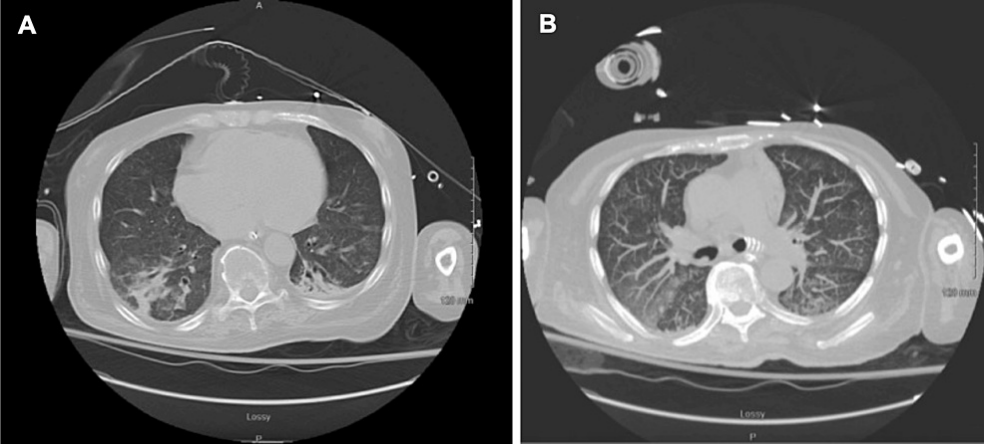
Tree-in-bud phenomenon seen on chest CT without contrast on day 25 of hospitalization.
